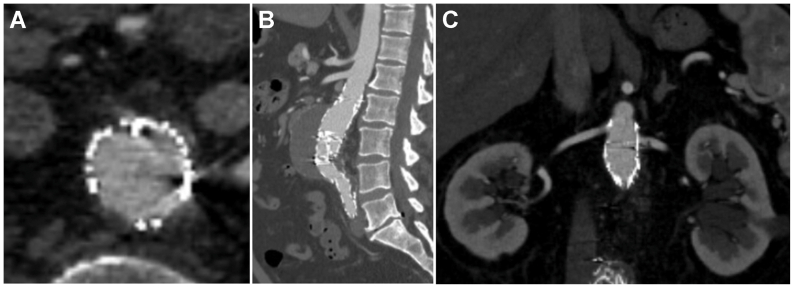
(A-C) One-month postoperative computed tomography (CT) angiography demonstrating a well-opposed endograft with no evidence of endoleaks.
